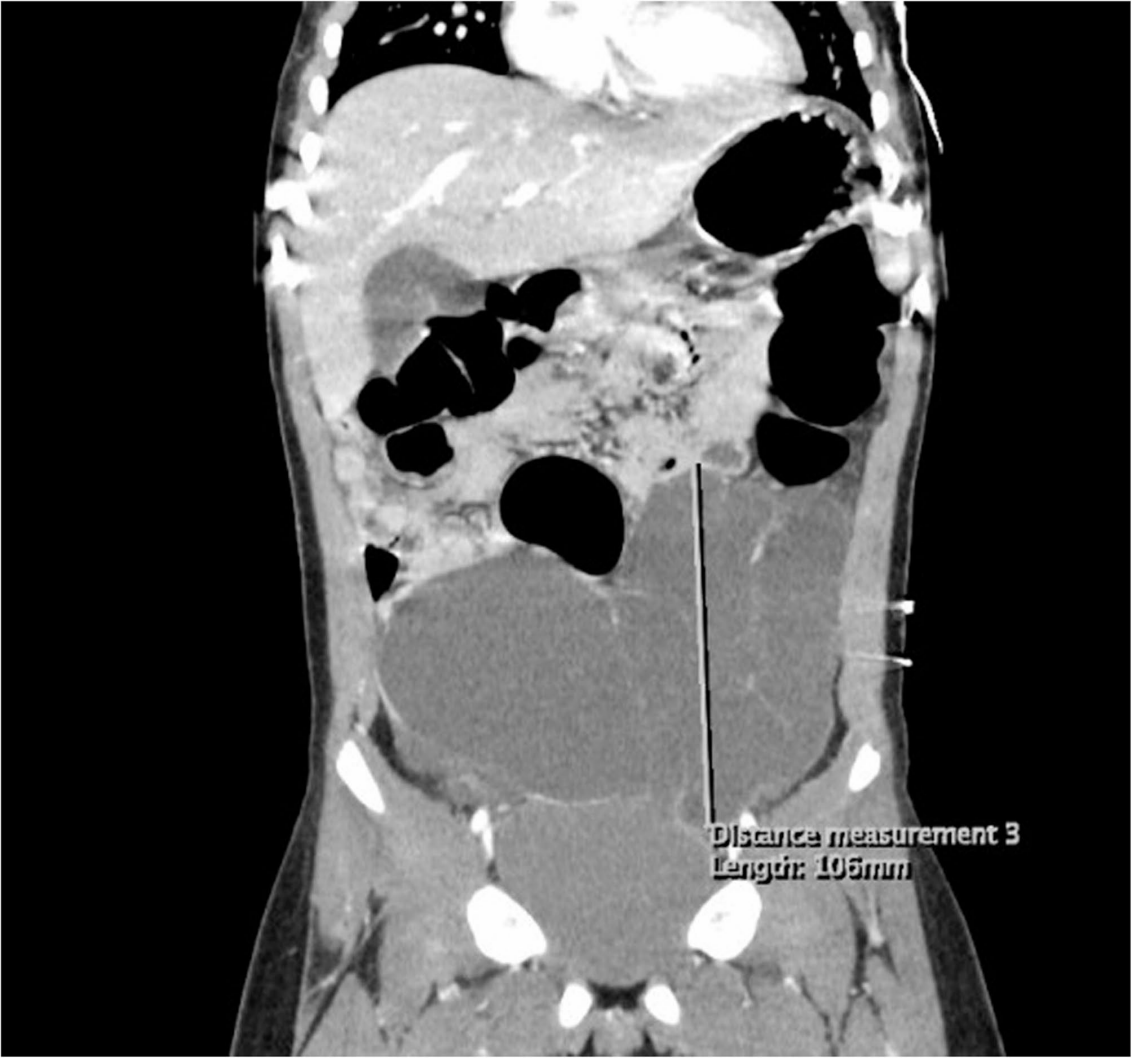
CT scan image of an abdominal cystic lymphangioma
